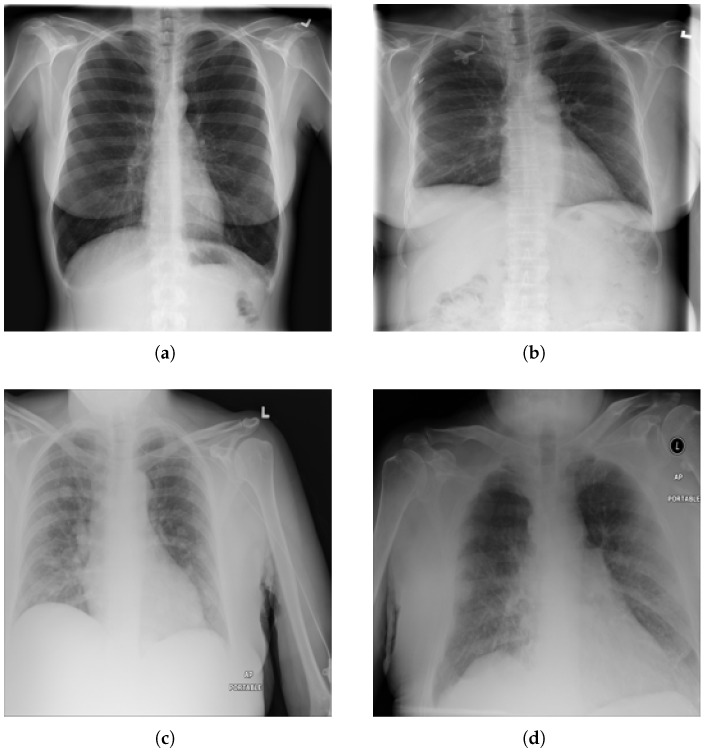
Examples from NIH chest X-ray14 datasets with labels: (a) No Finding, (b) Fibrosis. (c) Mass, and (d) Edema, Pleural Thickening, and Pneumonia.
